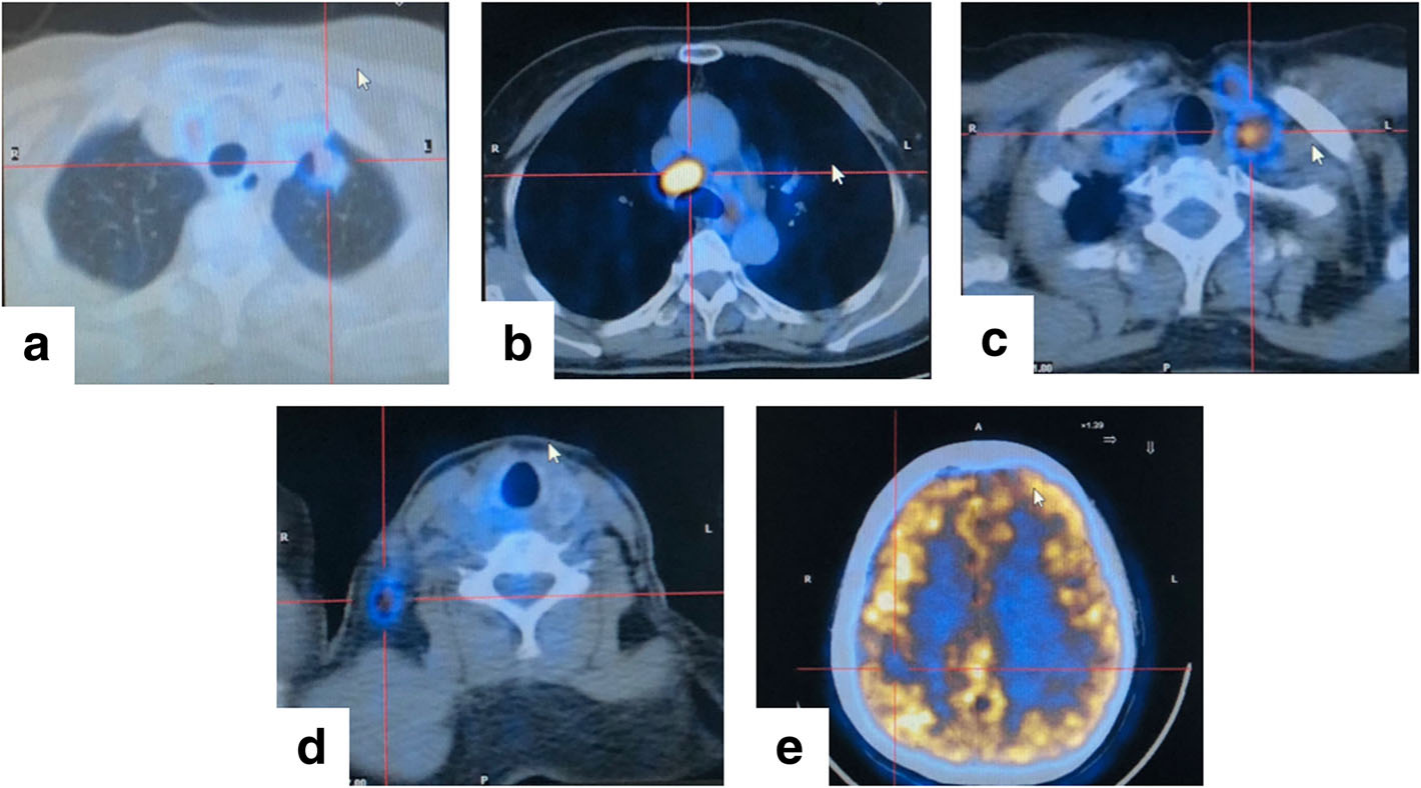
Positive finding by PET-CT scan at baseline. a Primary lung tumor, b–d metastatic lymph nodes, and (e) brain metastasis
